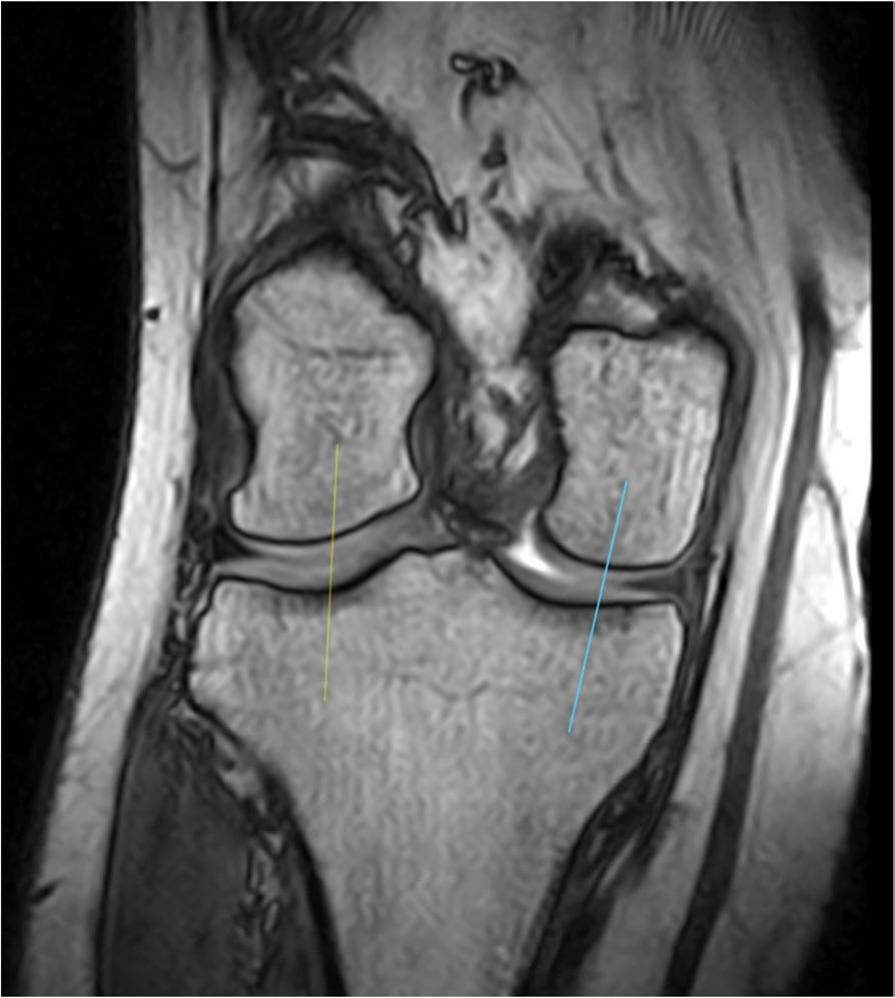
MRI cross-section with reference point for the measurement plane for the loading area of the cartilages of the medial and lateral femoral and tibial condyles (frontal plane)
